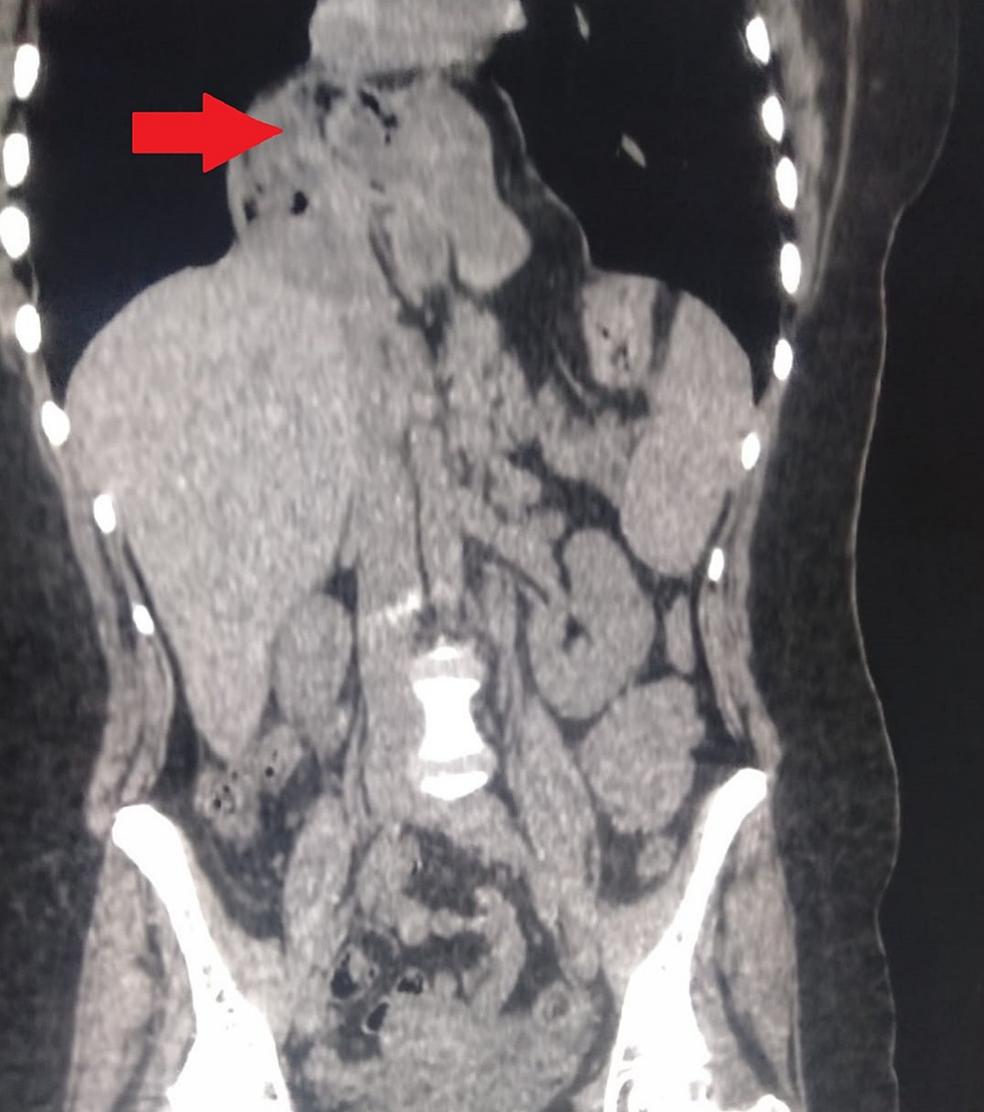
CT scan showing the paraesophageal hernia recurrence. Coronal scan showing the intrathoracic hernial sac (red arrow).
